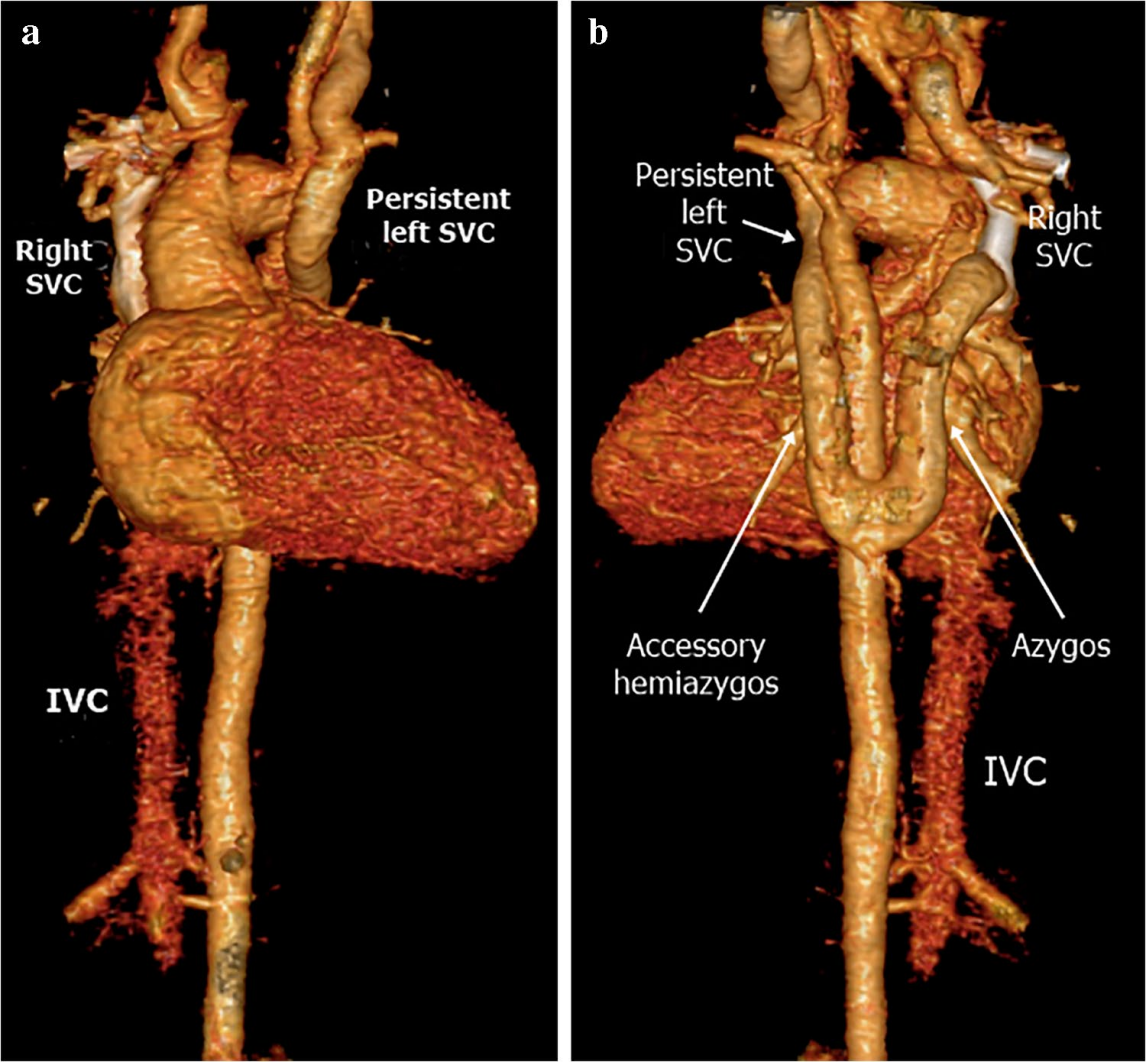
A persistent left superior vena cava (SVC) in a 1-year-old boy draining through an accessory hemiazygos vein to the azygos vein then the right SVC and right atrium. a, b There is no connection to the coronary sinus or the left atrium on anterior (a) or posterior (b) volume-rendered computed tomography images. Note the coarctation of aortic isthmus. *IVC* inferior vena cava
